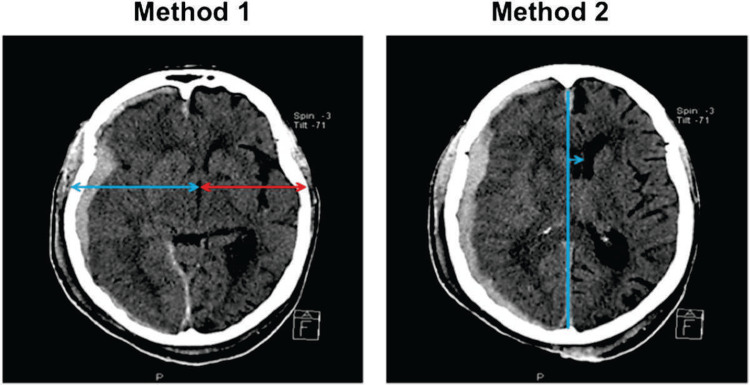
Method 1 shows a method of MLS measurement with a CT scan through the third ventricle, the same as TCS; Method 2 shows another method of measurement between the ideal median line and the septum pellucidum. MLS: midline shift; TCS: transcranial sonography
